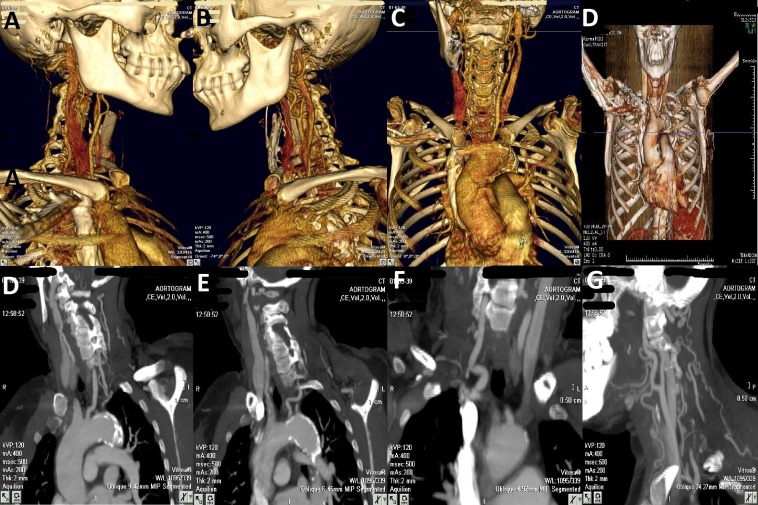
Computed tomography (CT) aortogram of chest [A-G] Severe stenosis of bilateral subclavian arteries with an appearance suggesting near occlusion. Mild to moderate stenosis of bilateral carotid arteries at the origin.
